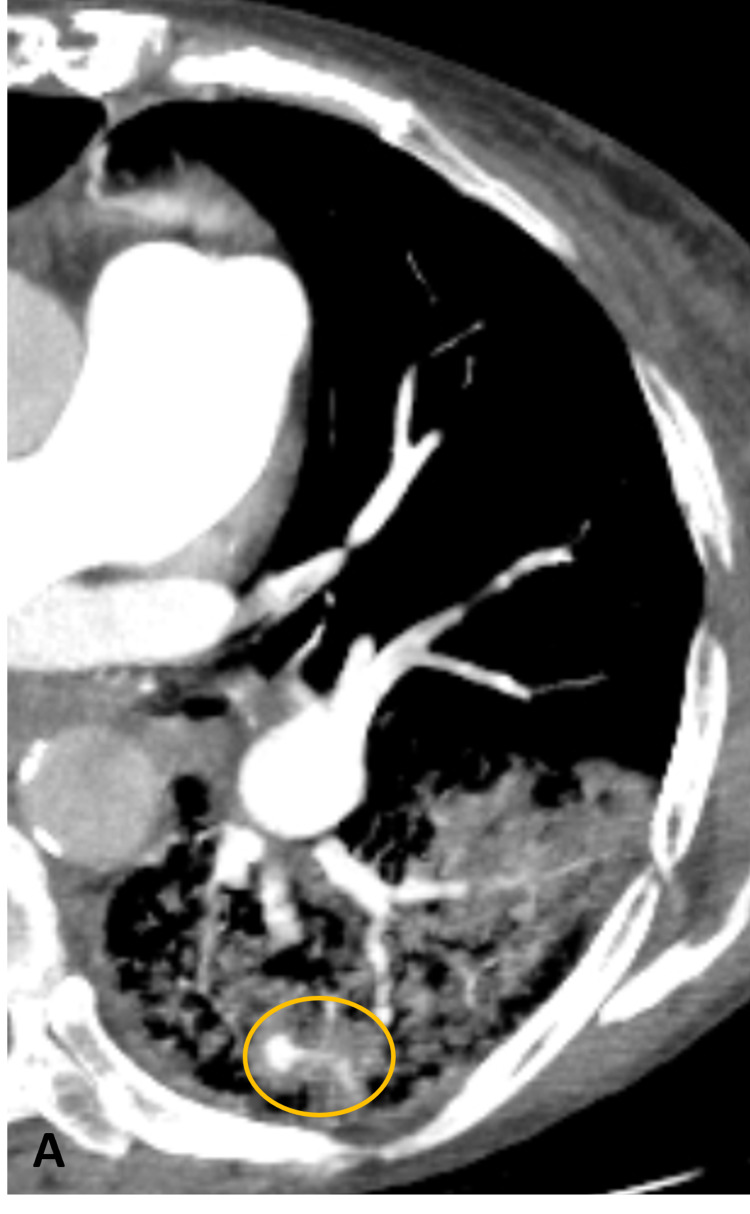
Peripheral pulmonary artery pseudoaneurysm. Five days post-biopsy CT image. Contrast-enhanced axial image at the level of the biopsy showing persistent alveolar haemorrhage and a subpleural peripheral pulmonary artery pseudoaneurysm (circle) at the site of the pulmonary nodule biopsy.
